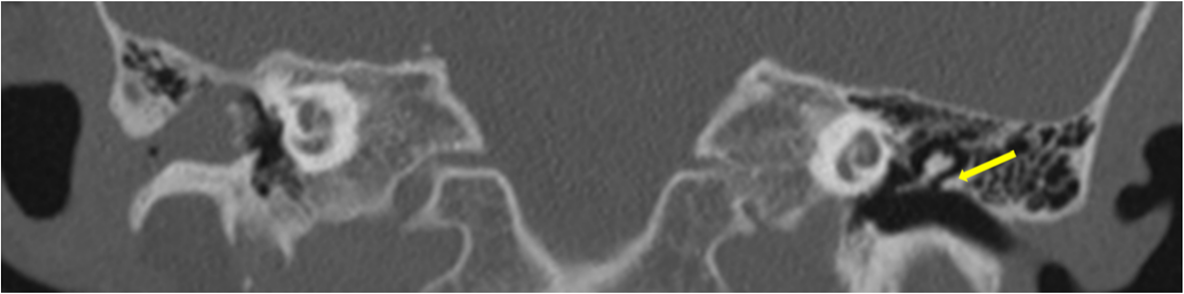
Coronal CT image showing normal scutum on the left side (arrow). It is seen as a point of superior attachment of the tympanic membrane. Scutum is eroded on the right side with soft tissue seen in the middle ear and external auditory canal
